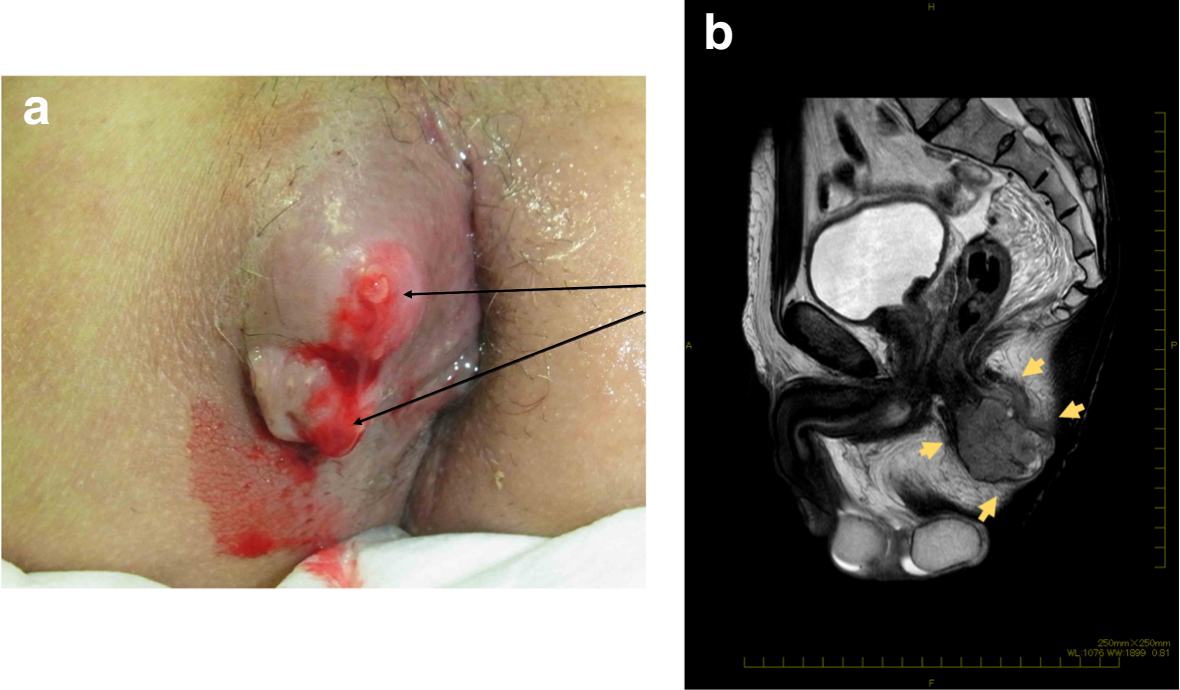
a An elastic hard tumor with two secondary openings (arrows) was detected at the right side of the anus. b MRI T2-weighted imaging revealed a heterogeneous high-intensity tumor of 4.5 cm in size at the perianal region (arrows)
